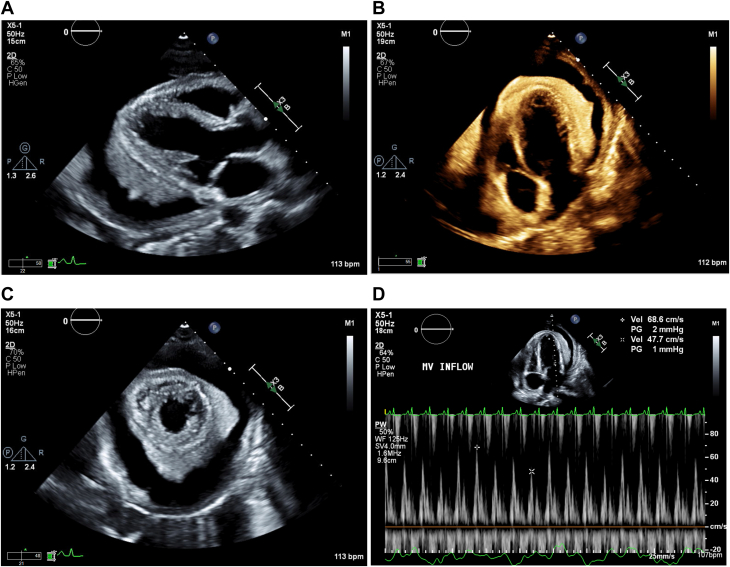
Initial Transthoracic Echocardiogram on the Patient's Presentation to the Hospital Multiple windows demonstrate the large pericardial effusion with fibrinous material collection adherent to the cardiac surface. (A) Parasternal long axis. (B) Apical 4-chamber view. (C) Parasternal short axis. (D) Mitral inflow demonstrating an abnormal respiratory variation of 30.47%, consistent with hemodynamic compromise.
